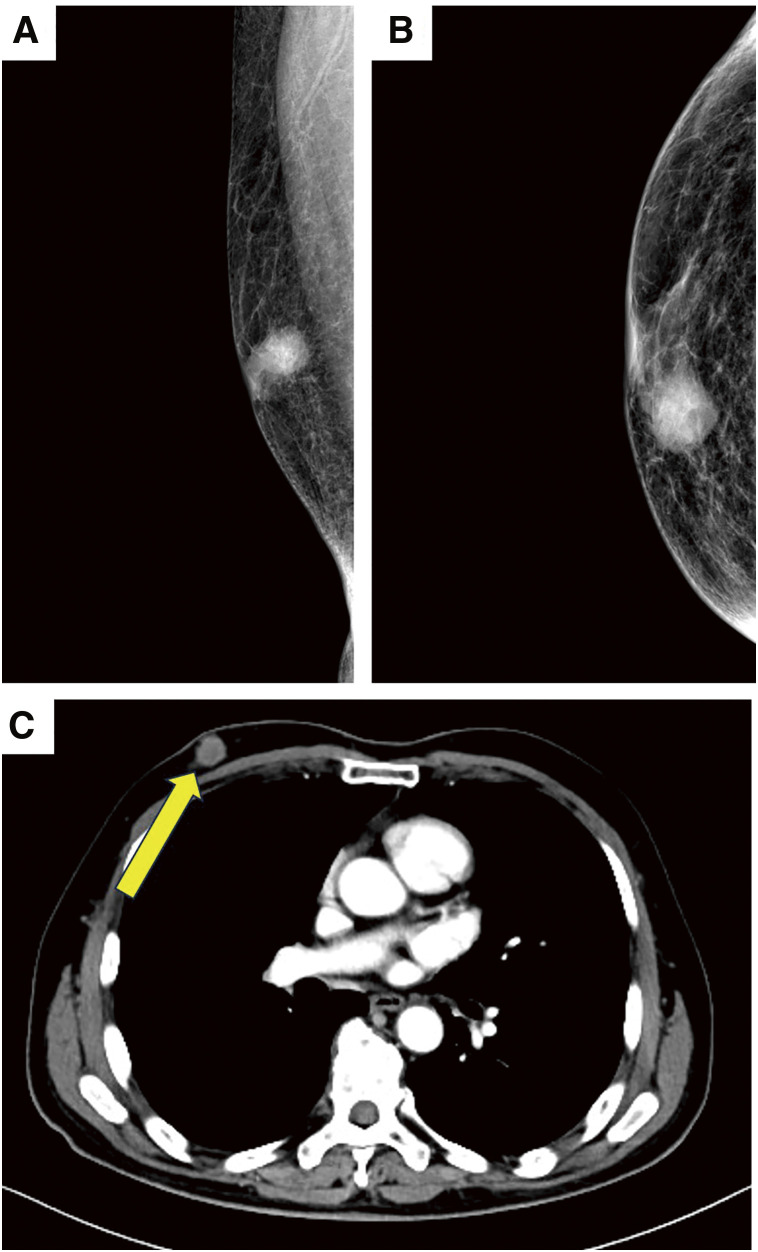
Mammogram results. Mammography shows a micro-lobulated and round mass without calcification in the right craniocaudal inner (A) and mediolateral-oblique middle areas (B). (C) Contrast-enhanced computed tomography reveals a solitary mass in the right breast (arrow).
